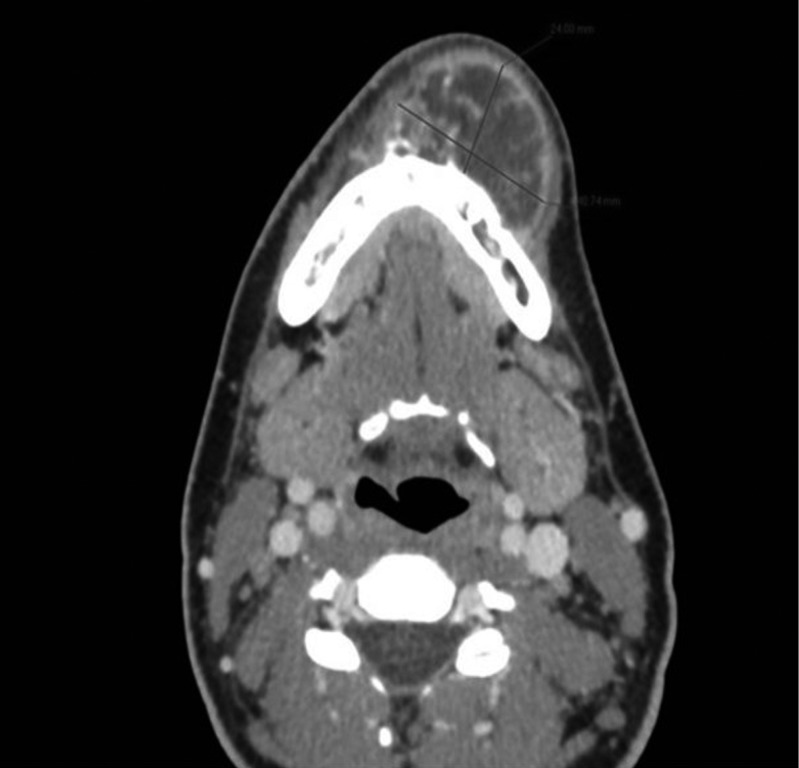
Sagittal CT images of mandibular chondrosarcoma.
